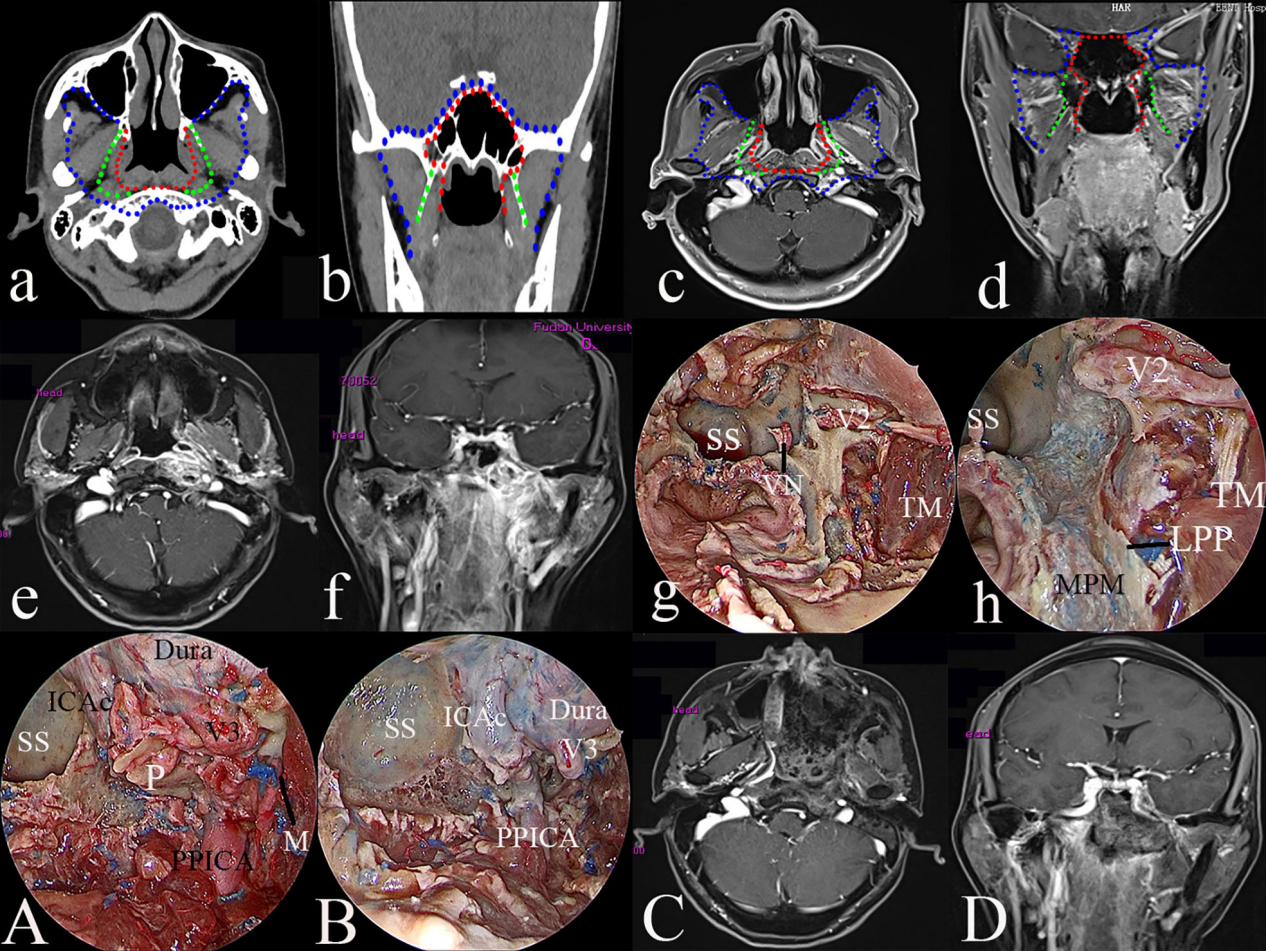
The greatest extent of resection in Type III transnasal endoscopic nasopharyngectomy (blue dot line in a–d), pre-operative magnetic resonance imaging (MRI) of rT3 nasopharyngeal carcinoma (NPC) (e, f); exposure of the key landmarks in Type III TEN (g, h, A, B); and post-operative MRI (C, D). ICAc, clival internal carotid artery; LPP, the lateral pterygoid plate; MPM, medial pterygoid muscle; M, middle meningeal artery; P, petrous bone; PPICA, parapharyngeal internal carotid artery; SS, sphenoid sinus; TM, temporal muscle; VN, vidian nerve; V2, maxillary nerve; V3, mandibular nerve.
